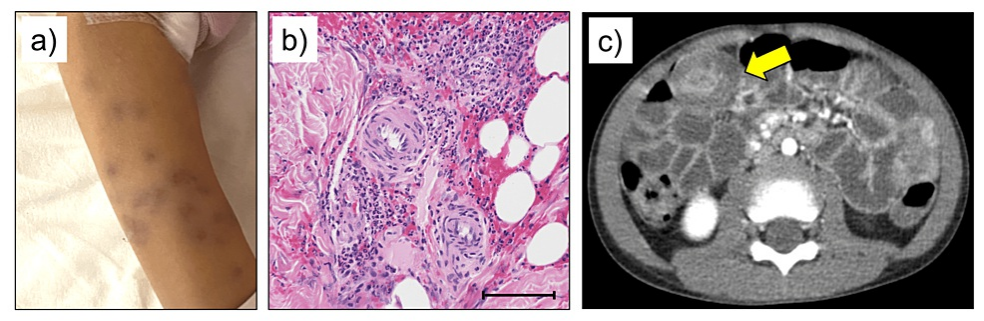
Clinical image of our patient (IgAV associated with MEFV gene mutation). a) Purpura in the lower extremities. b) Skin biopsy of the purpura revealed leukocytoclastic vasculitis (scale bar: 100 μm). However, IgA deposition could not be observed. c) Abdominal CT scan performed at day 23 revealed target signs indicating intussusception (yellow arrow). IgAV, IgA vasculitis; MEFV gene, the familial Mediterranean fever gene
